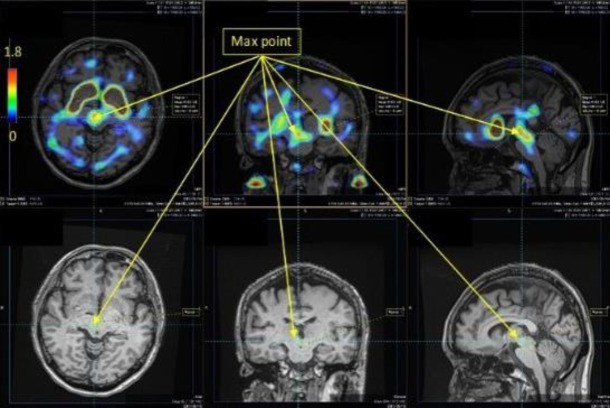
An example of a specific binding ratio (SBR) image of the brain stem. Serotonin transporter accumulation is localised in the brain stem, as revealed by overlapping the magnetic resonance image, and the maximum SBR value is 1.8. This is lower than the SBR maximum value in the striatum
